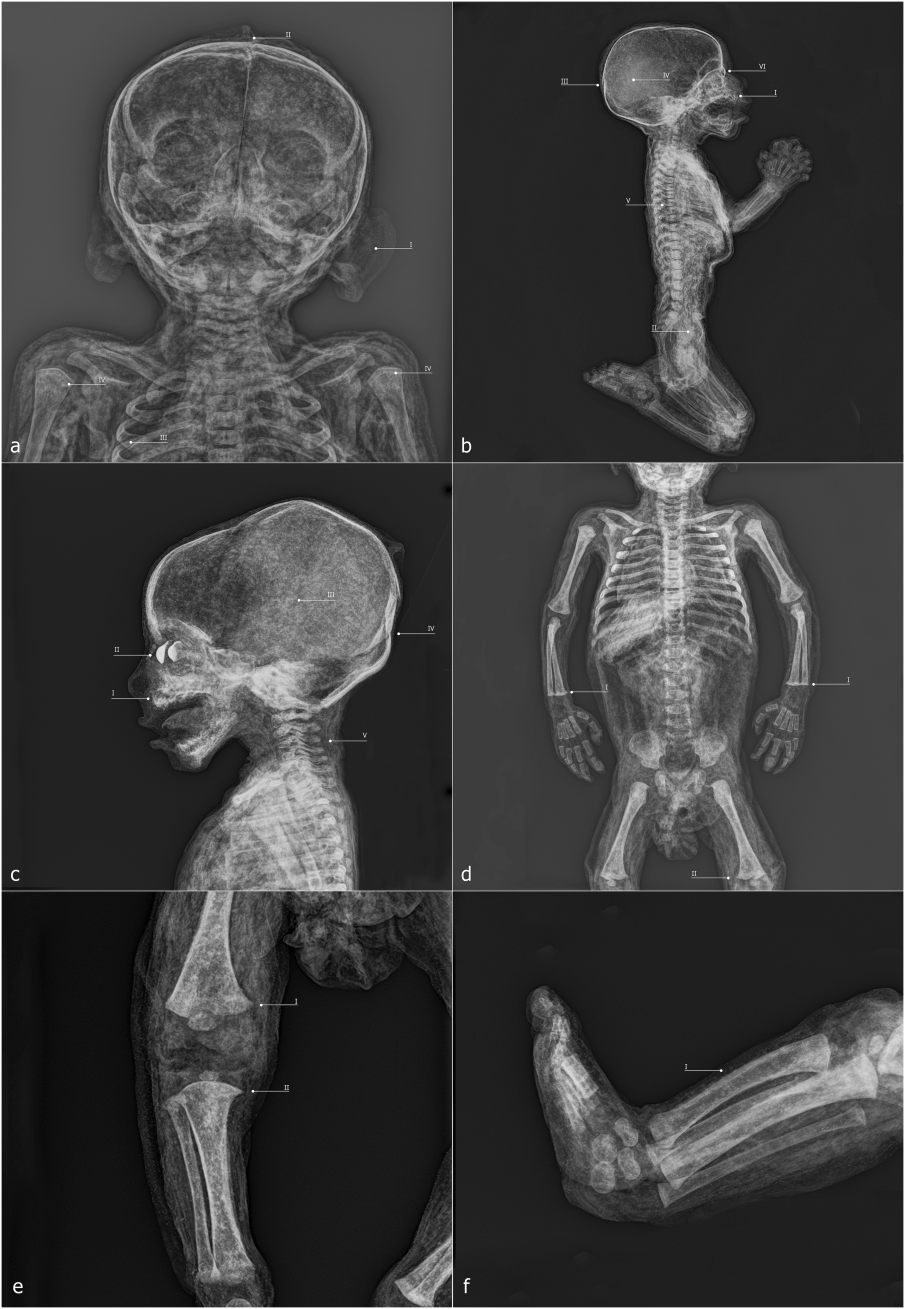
ID1—radiographic images: anteroposterior view of the head and shoulders (a); full-body laterolateral view (b). ID2—radiographic images: laterolateral view of the skull and shoulders (c); anteroposterior view of the trunk and upper limbs (D); anteroposterior view of the right leg (e); laterolateral view of the legs and feet (f).
